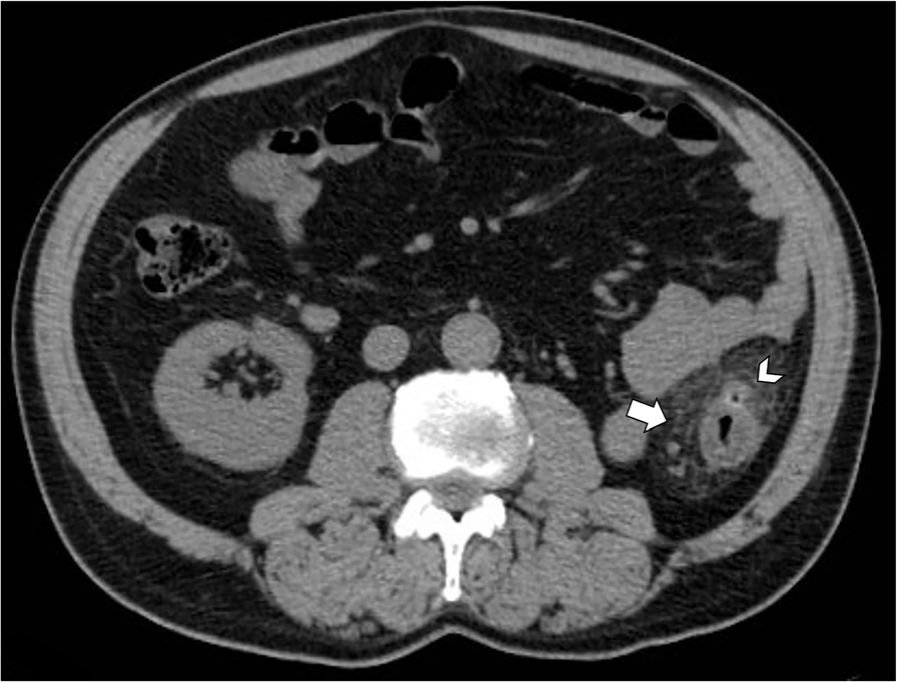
Acute diverticulitis in a 55-year-old man. Axial non-contrast CT image shows segmental thickening of descending colon, with pericolonic fat stranding (arrow). An inflamed diverticulum is identified abutting the anterior colonic wall (arrowhead)
